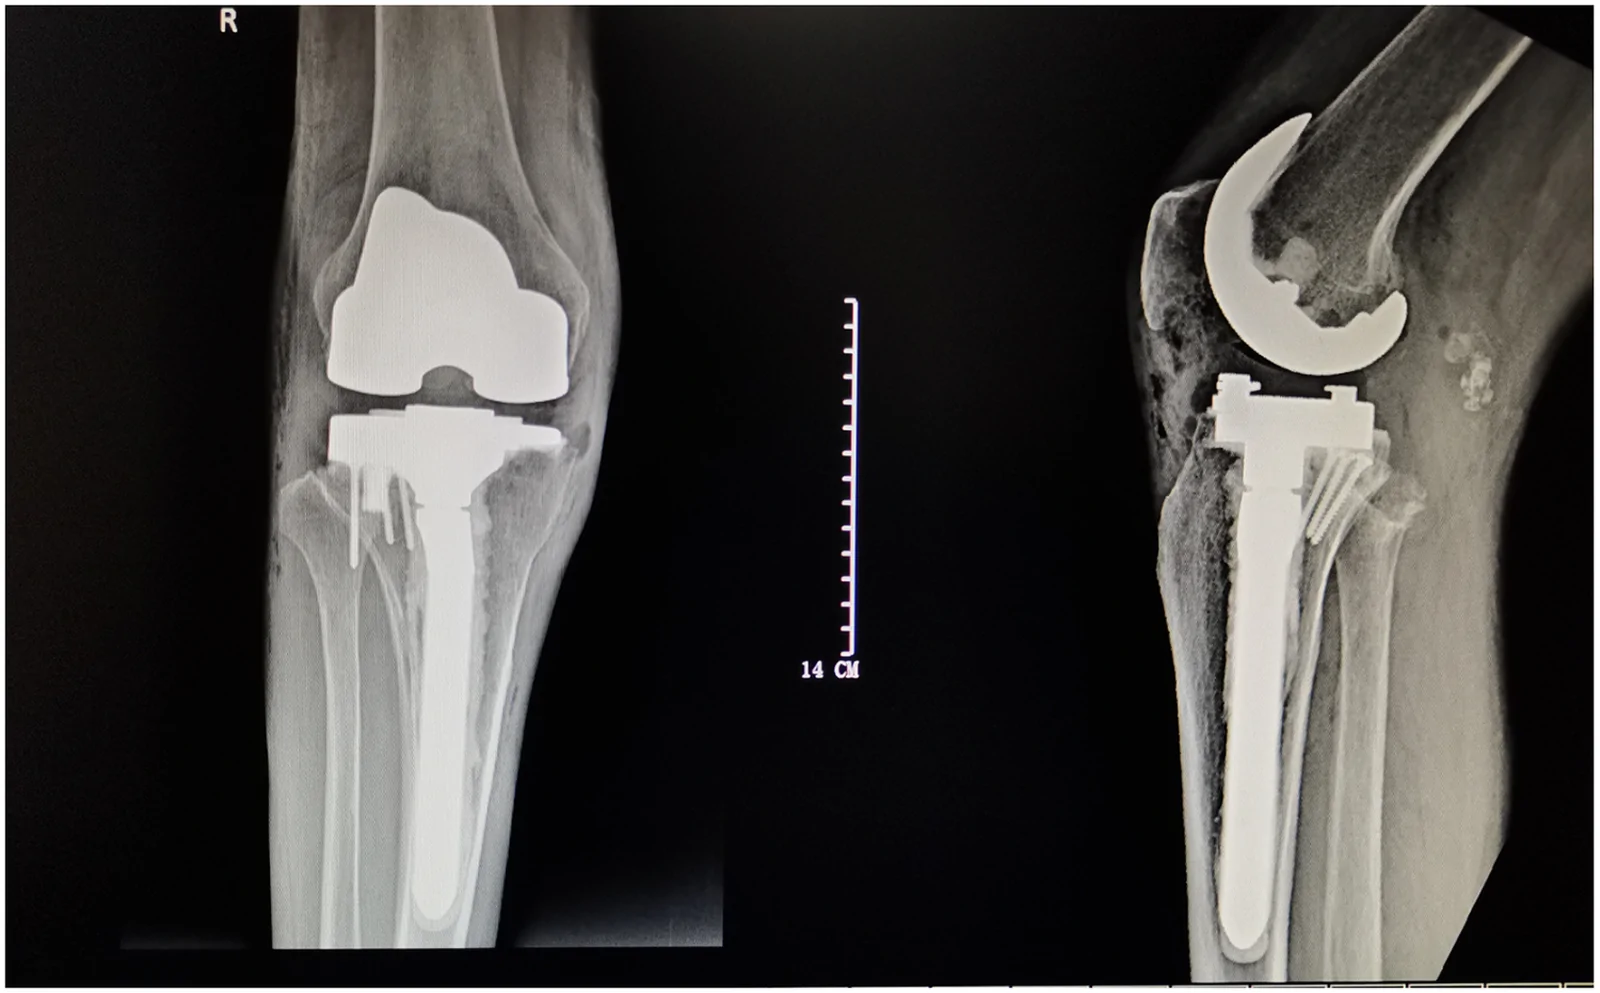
Postoperative anteroposterior and lateral view radiograph of the right knee.
